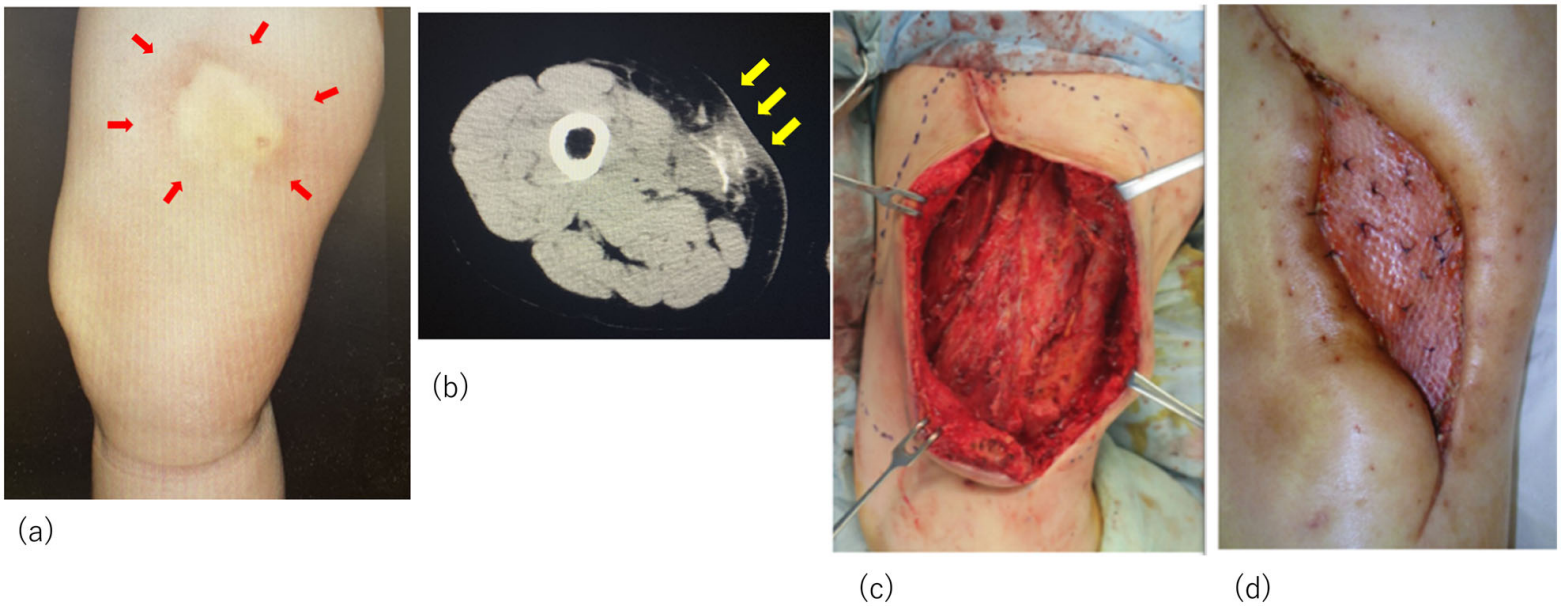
Initial examination and surgery of the patient. (a) The initial lesion of the thigh; arrows indicate the indurated nodule. (b) Computed tomography images indicating calcification and inflammation. Arrows indicate the calcification. (c) Intraoperative view of the thigh after radical debridement. (d) Successful placement of the skin graft.
